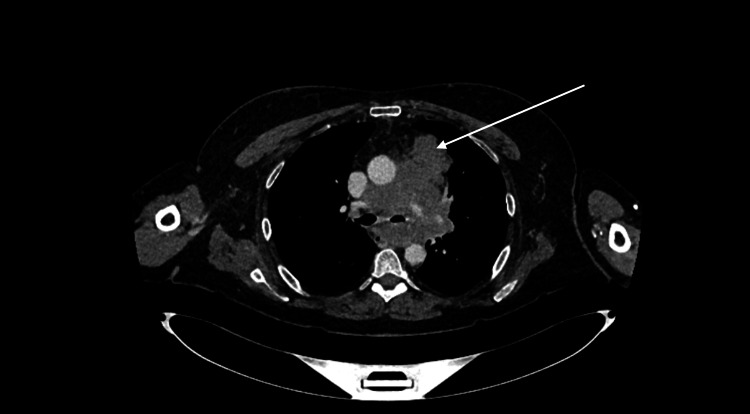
CT neck demonstrating progressive mediastinal lymphadenopathy labeled by the white arrow
